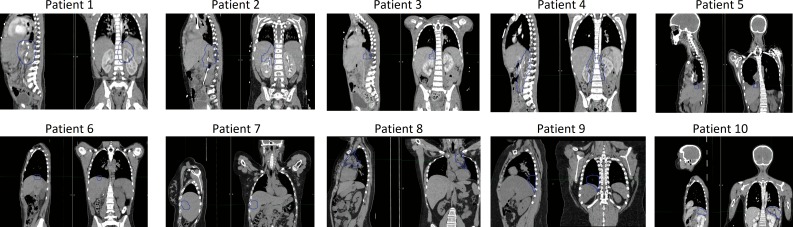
Sagittal and coronal computed tomography scans of the 10 patients with the internal target volume contours overlaid.
